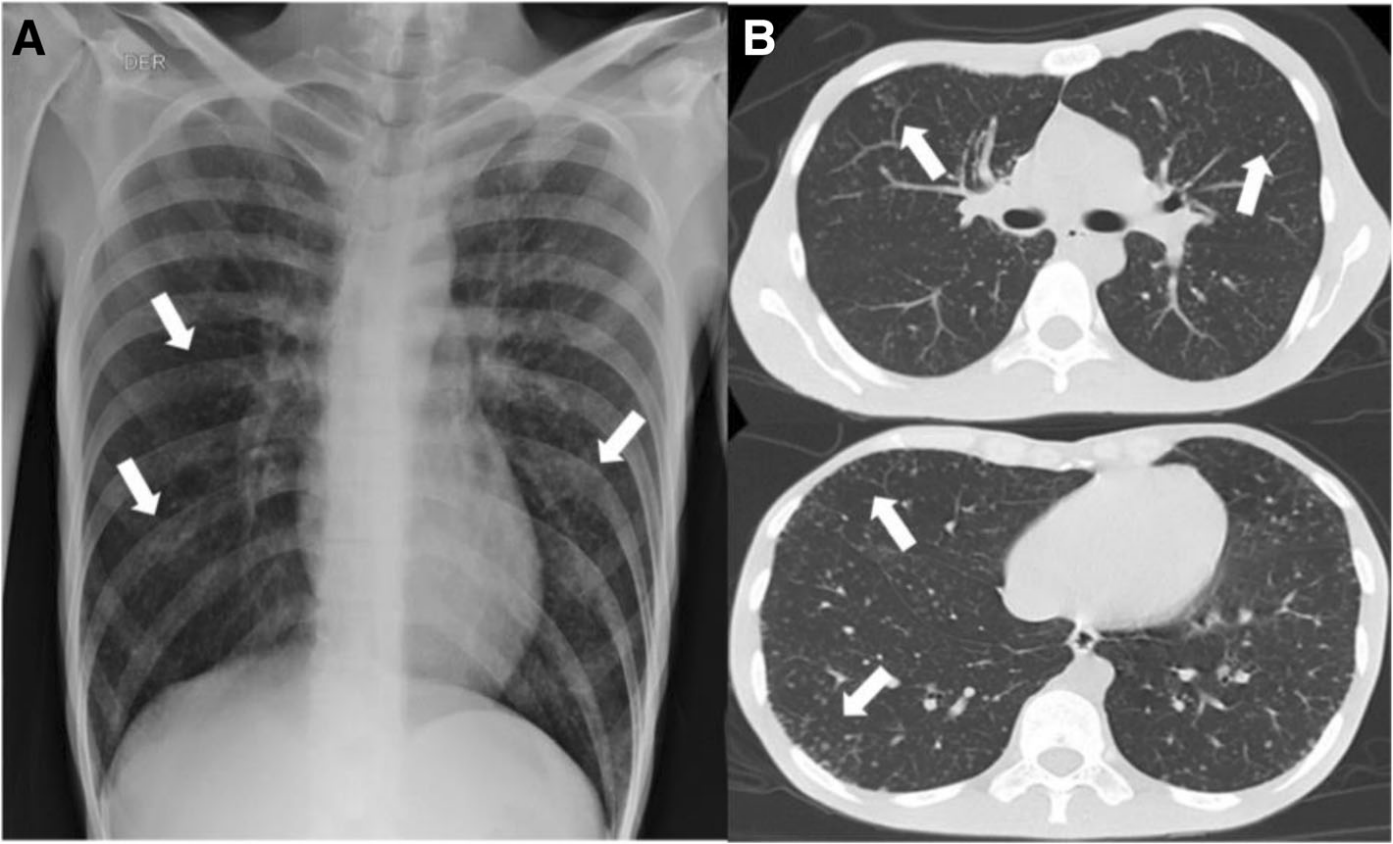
Patient images showing random distribution of micro-nodular opacities. a. Chest X-ray. b. Thorax CT scan
